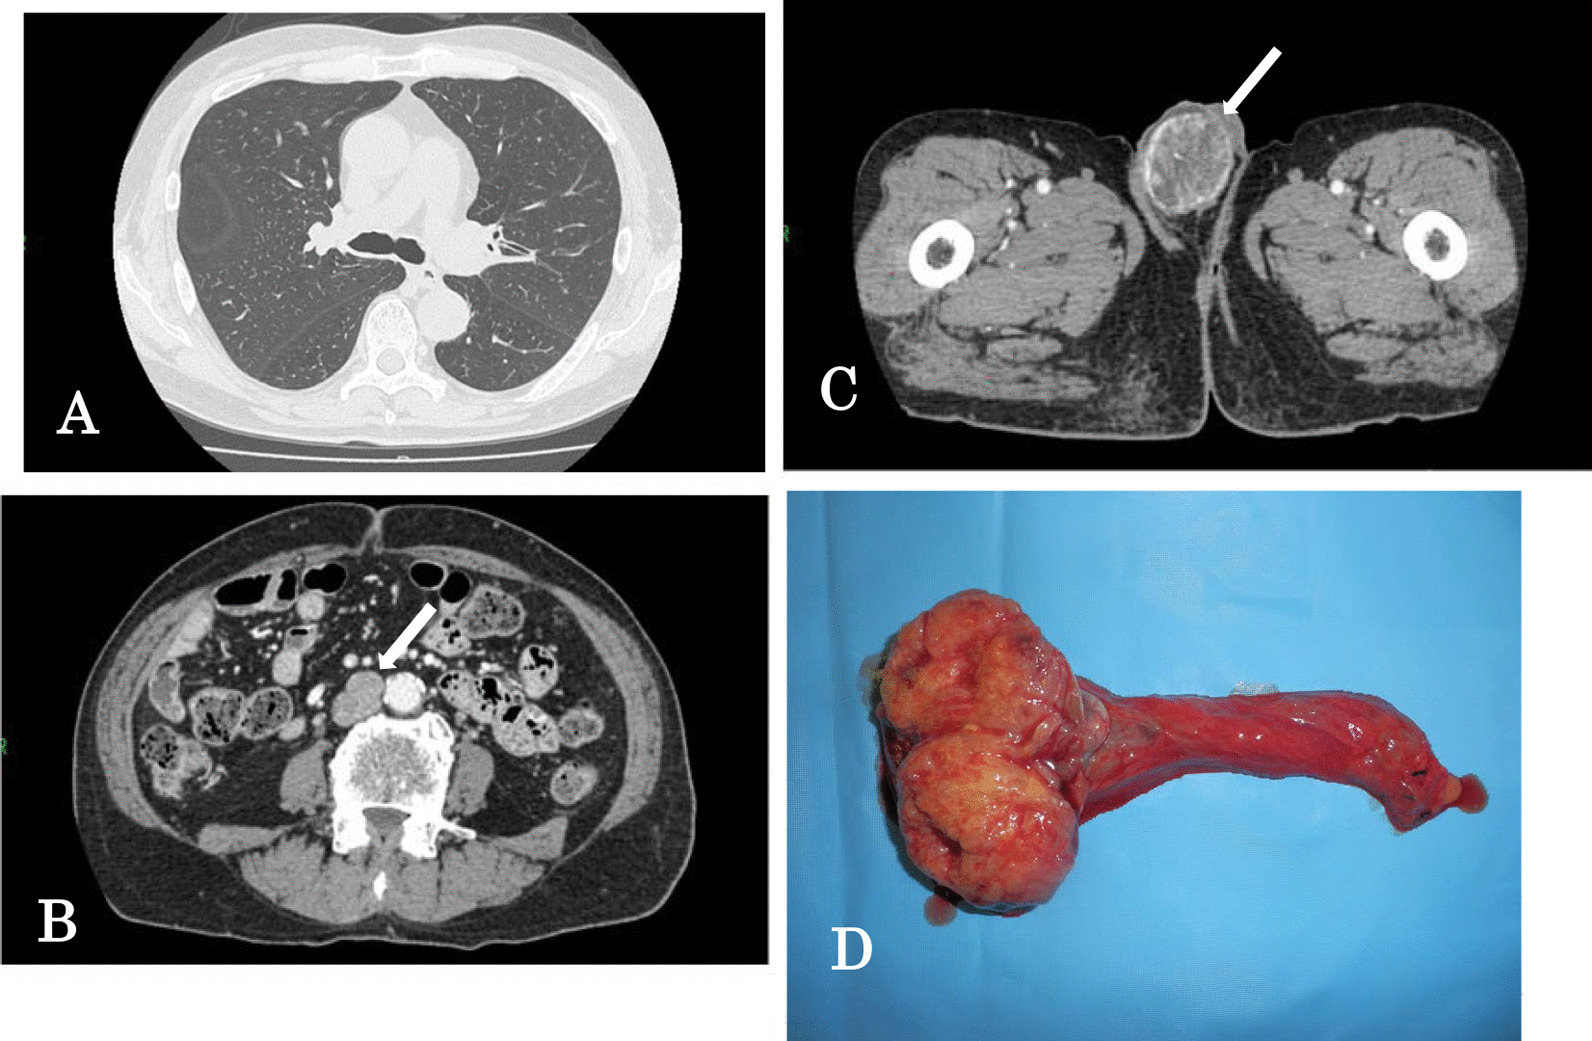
Lung CT showed no evidence of any coin lesions (A). Contrast-enhanced CT showed enlargements of the para-aortic lymph node (B, 18 × 16 mm^2^, white arrow), and a 50 × 45 mm^3^ mass with uneven contents was found in the right testicle (C, white arrow). The diagnosis was a testicular tumor (cT1, N1, M0, S0, and TNM stage IIA). The resected testicular tumor was 55 × 45 × 40 mm^3^ in size and 63 g in weight. The cut surface was yellowish-white with bleeding and solid, and the testicular tumor was localized in the testicle (D)
